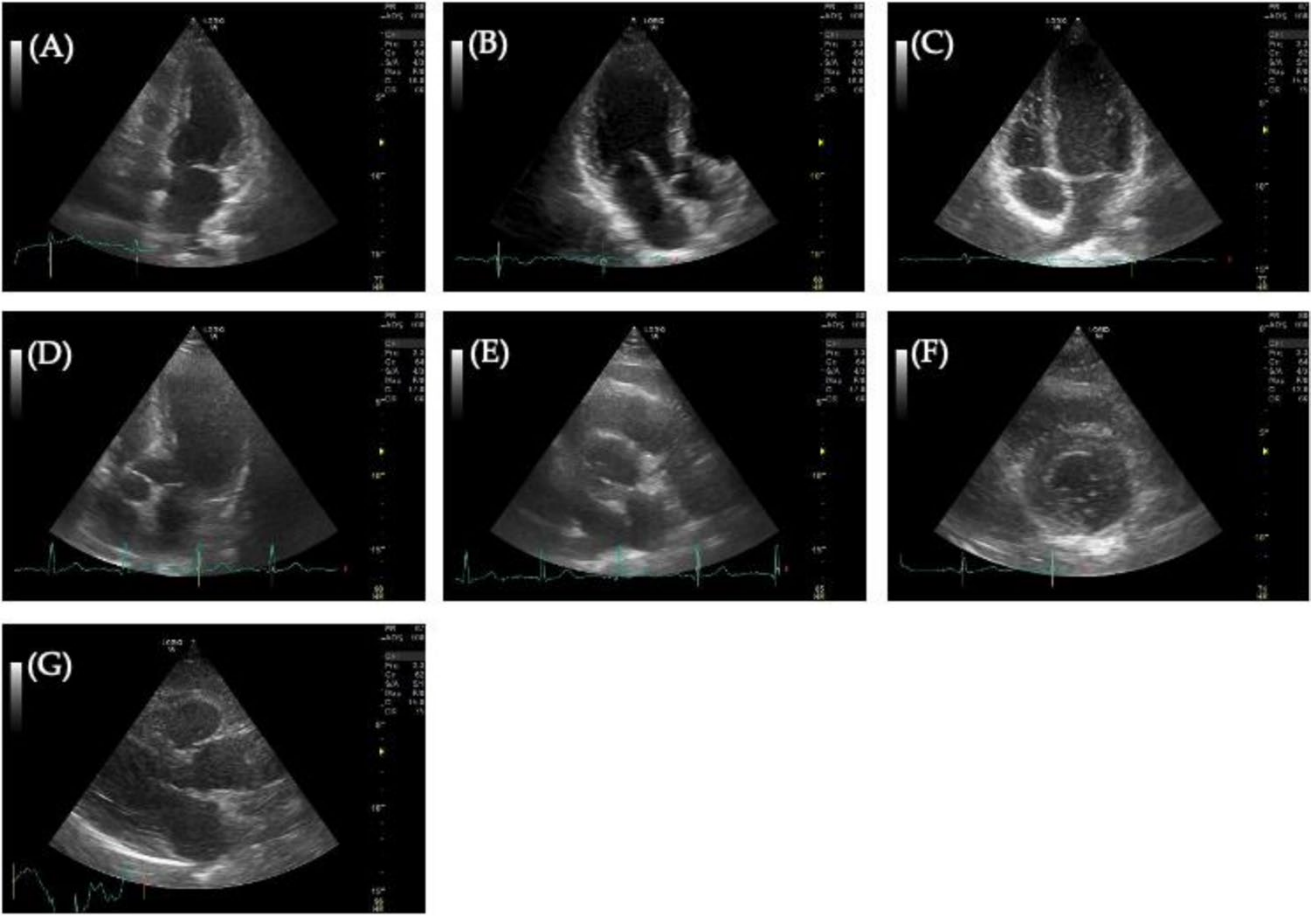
Different types of heart view on Echocardiography: (A) Apical 2-chamber view; (B) Apical 3-chamber view; (C) Apical 4-chamber view; (D) Apical 5-chamber view; (E) Parasternal short-axis view; (F) Parasternal short-axis view of the left ventricle; (G) Parasternal long-axis view
